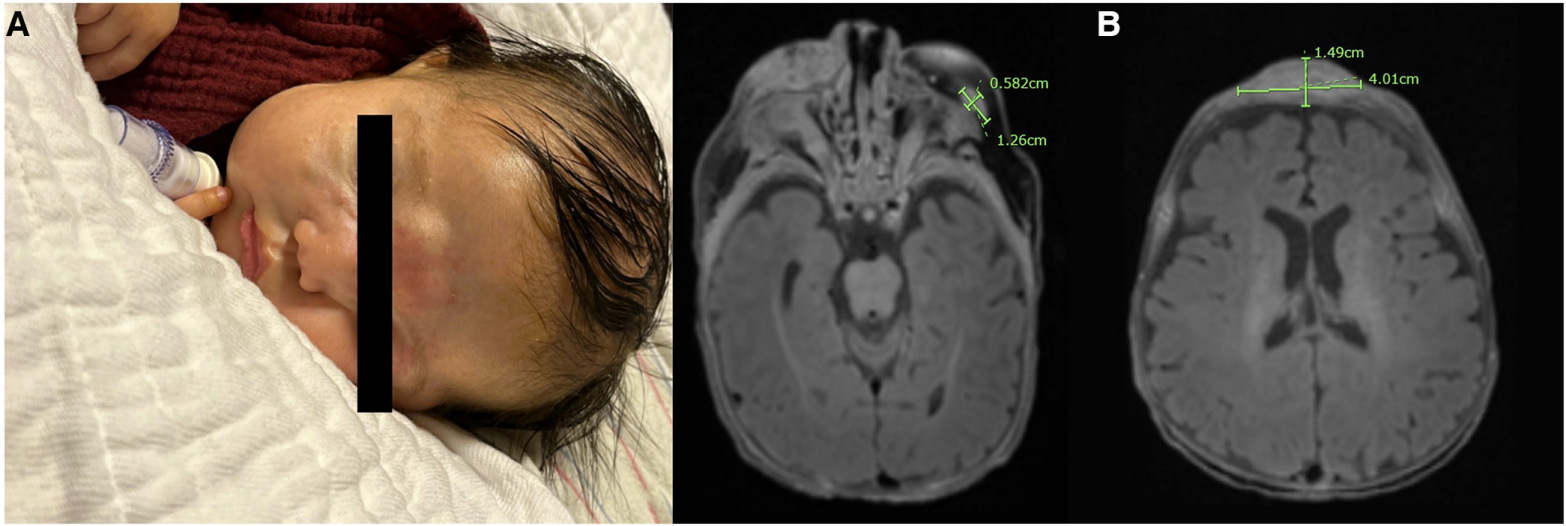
(A) Image of the infant's face taken after completion of cycle 1 with a notable reduction in the size of the facial lesions; the infant was able to fully open her eyes spontaneously at this point in therapy. (B) MRI of the brain taken prior to starting salvage therapy with an almost complete resolution of intracranial metastases and a notable reduction in the size of prior tumors.
